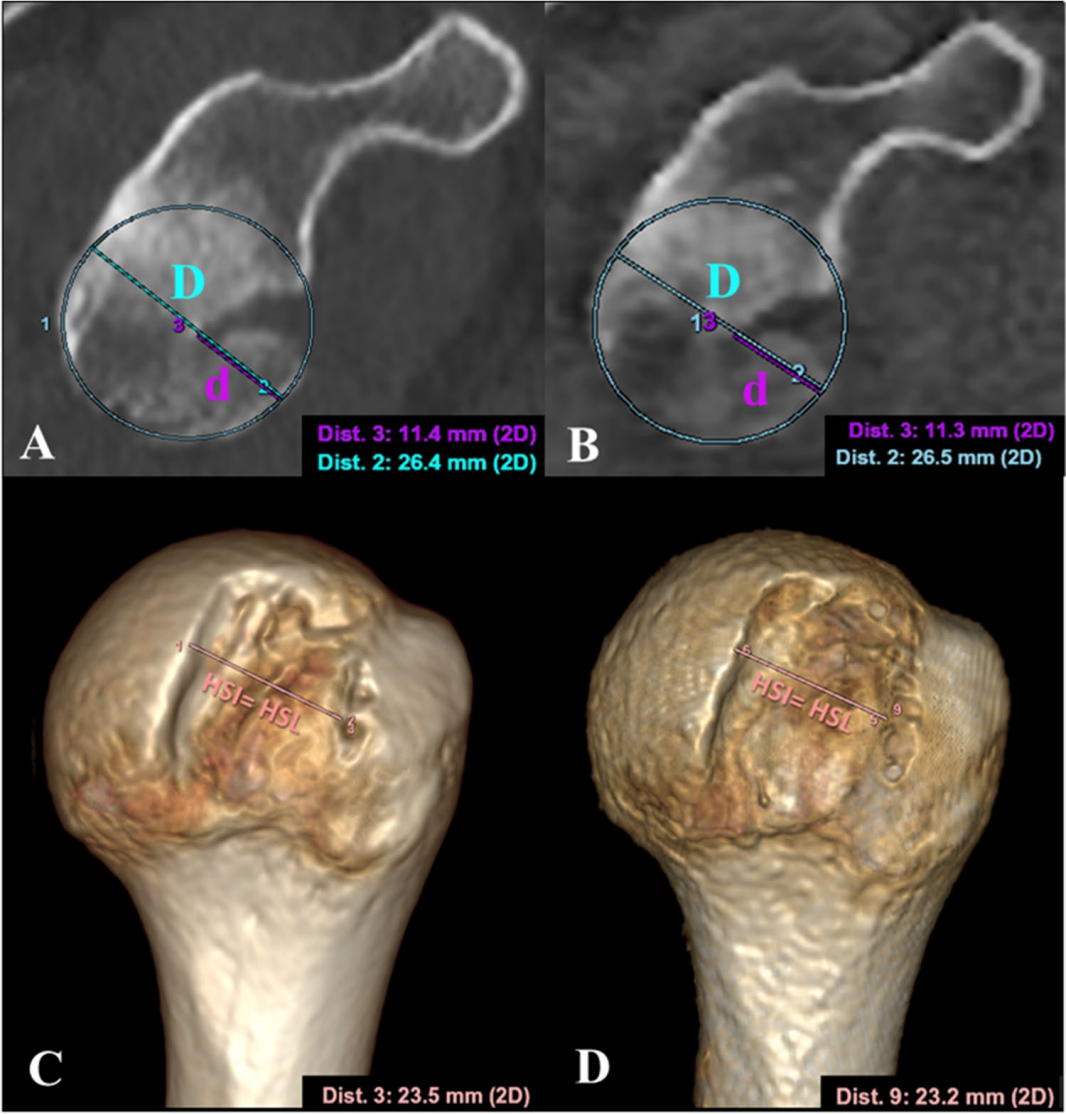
Bipolar bone loss measurement. GT measurement through the best-fit circle on sagittal en face glenoid view on CT (A) and bone image from DL ZTE (B). Both diameter (*D*) and glenoid bone loss (*d*) are shown in blue and purple respectively. Leading to a GT of 10.51 mm for CT and 10.70 mm for DL ZTE. HSI measurement on 3D volumes, CT (C) and DL ZTE (D). In this case, there is no BB, so all the HSI corresponds to the HSL, 23.5 mm for CT (C) and 23.2 mm for ZTE (D)
